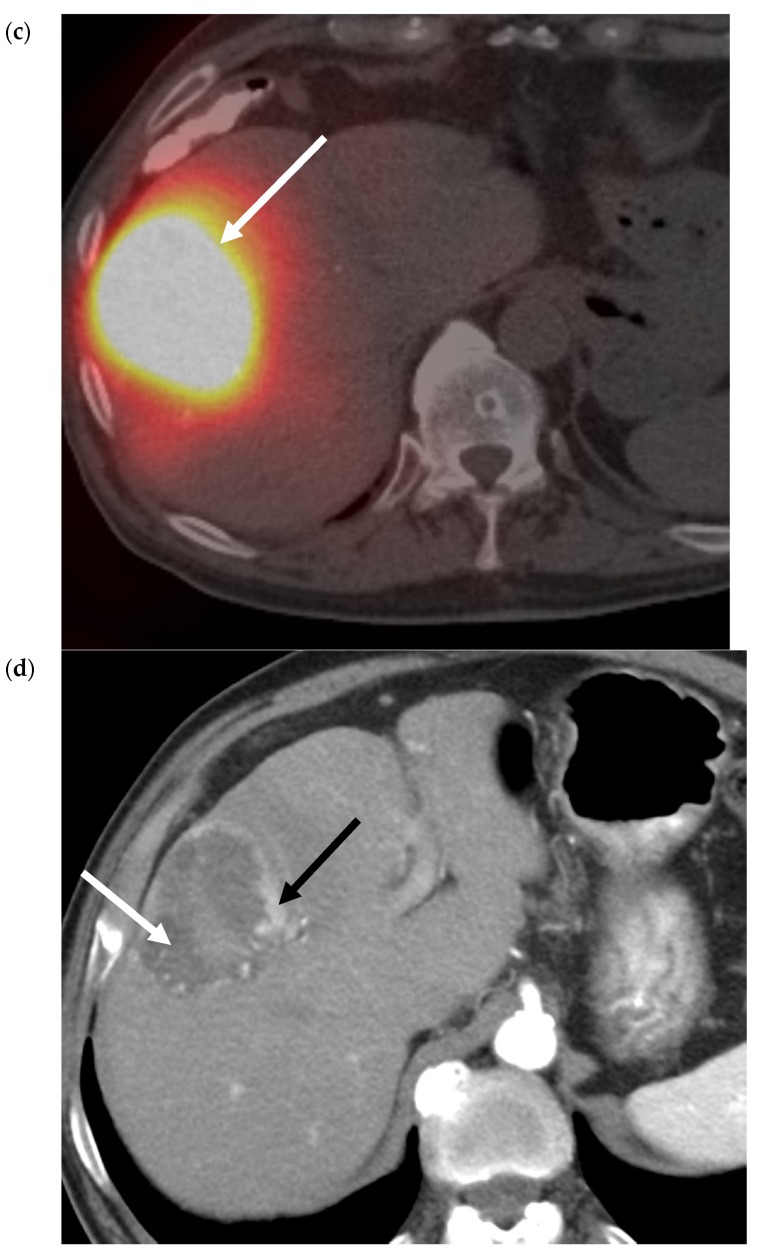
90Y Radiation Segmentectomy for Solitary HCC. (a) Pre-procedure triphasic contrast-enhanced CT in the hepatic arterial phase. Mass (white arrow) with arterial hyperenhancement in the anterior sector of the right lobe of the liver, consistent with solitary HCC. (b). Digital subtraction angiogram of the anterior division of the right hepatic artery prior to administration of glass 90Y microspheres. Mass (white arrow) is supplied by the anterior division of the right hepatic artery (black arrow). (c) SPECT image immediately post-90Y radioembolization. High activity of 90Y microspheres in the liver tumor (white arrow) is demonstrated without evidence of nontarget delivery. (d) Post-procedure triphasic contrast-enhanced CT in the arterial phase performed 6 weeks post-treatment. Marked decrease in arterial hyperenhancement of the anterior right hepatic tumor. Overall, the mass is hypoenhancing (white arrow) due to the treatment effect, with a rim and few areas of residual enhancement (black arrow).
